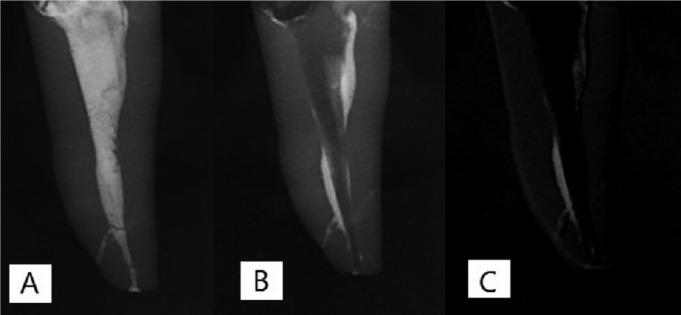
A) Tooth prior to root canal preparation B) Tooth after root canal preparation C) Digital subtraction radiograph.
